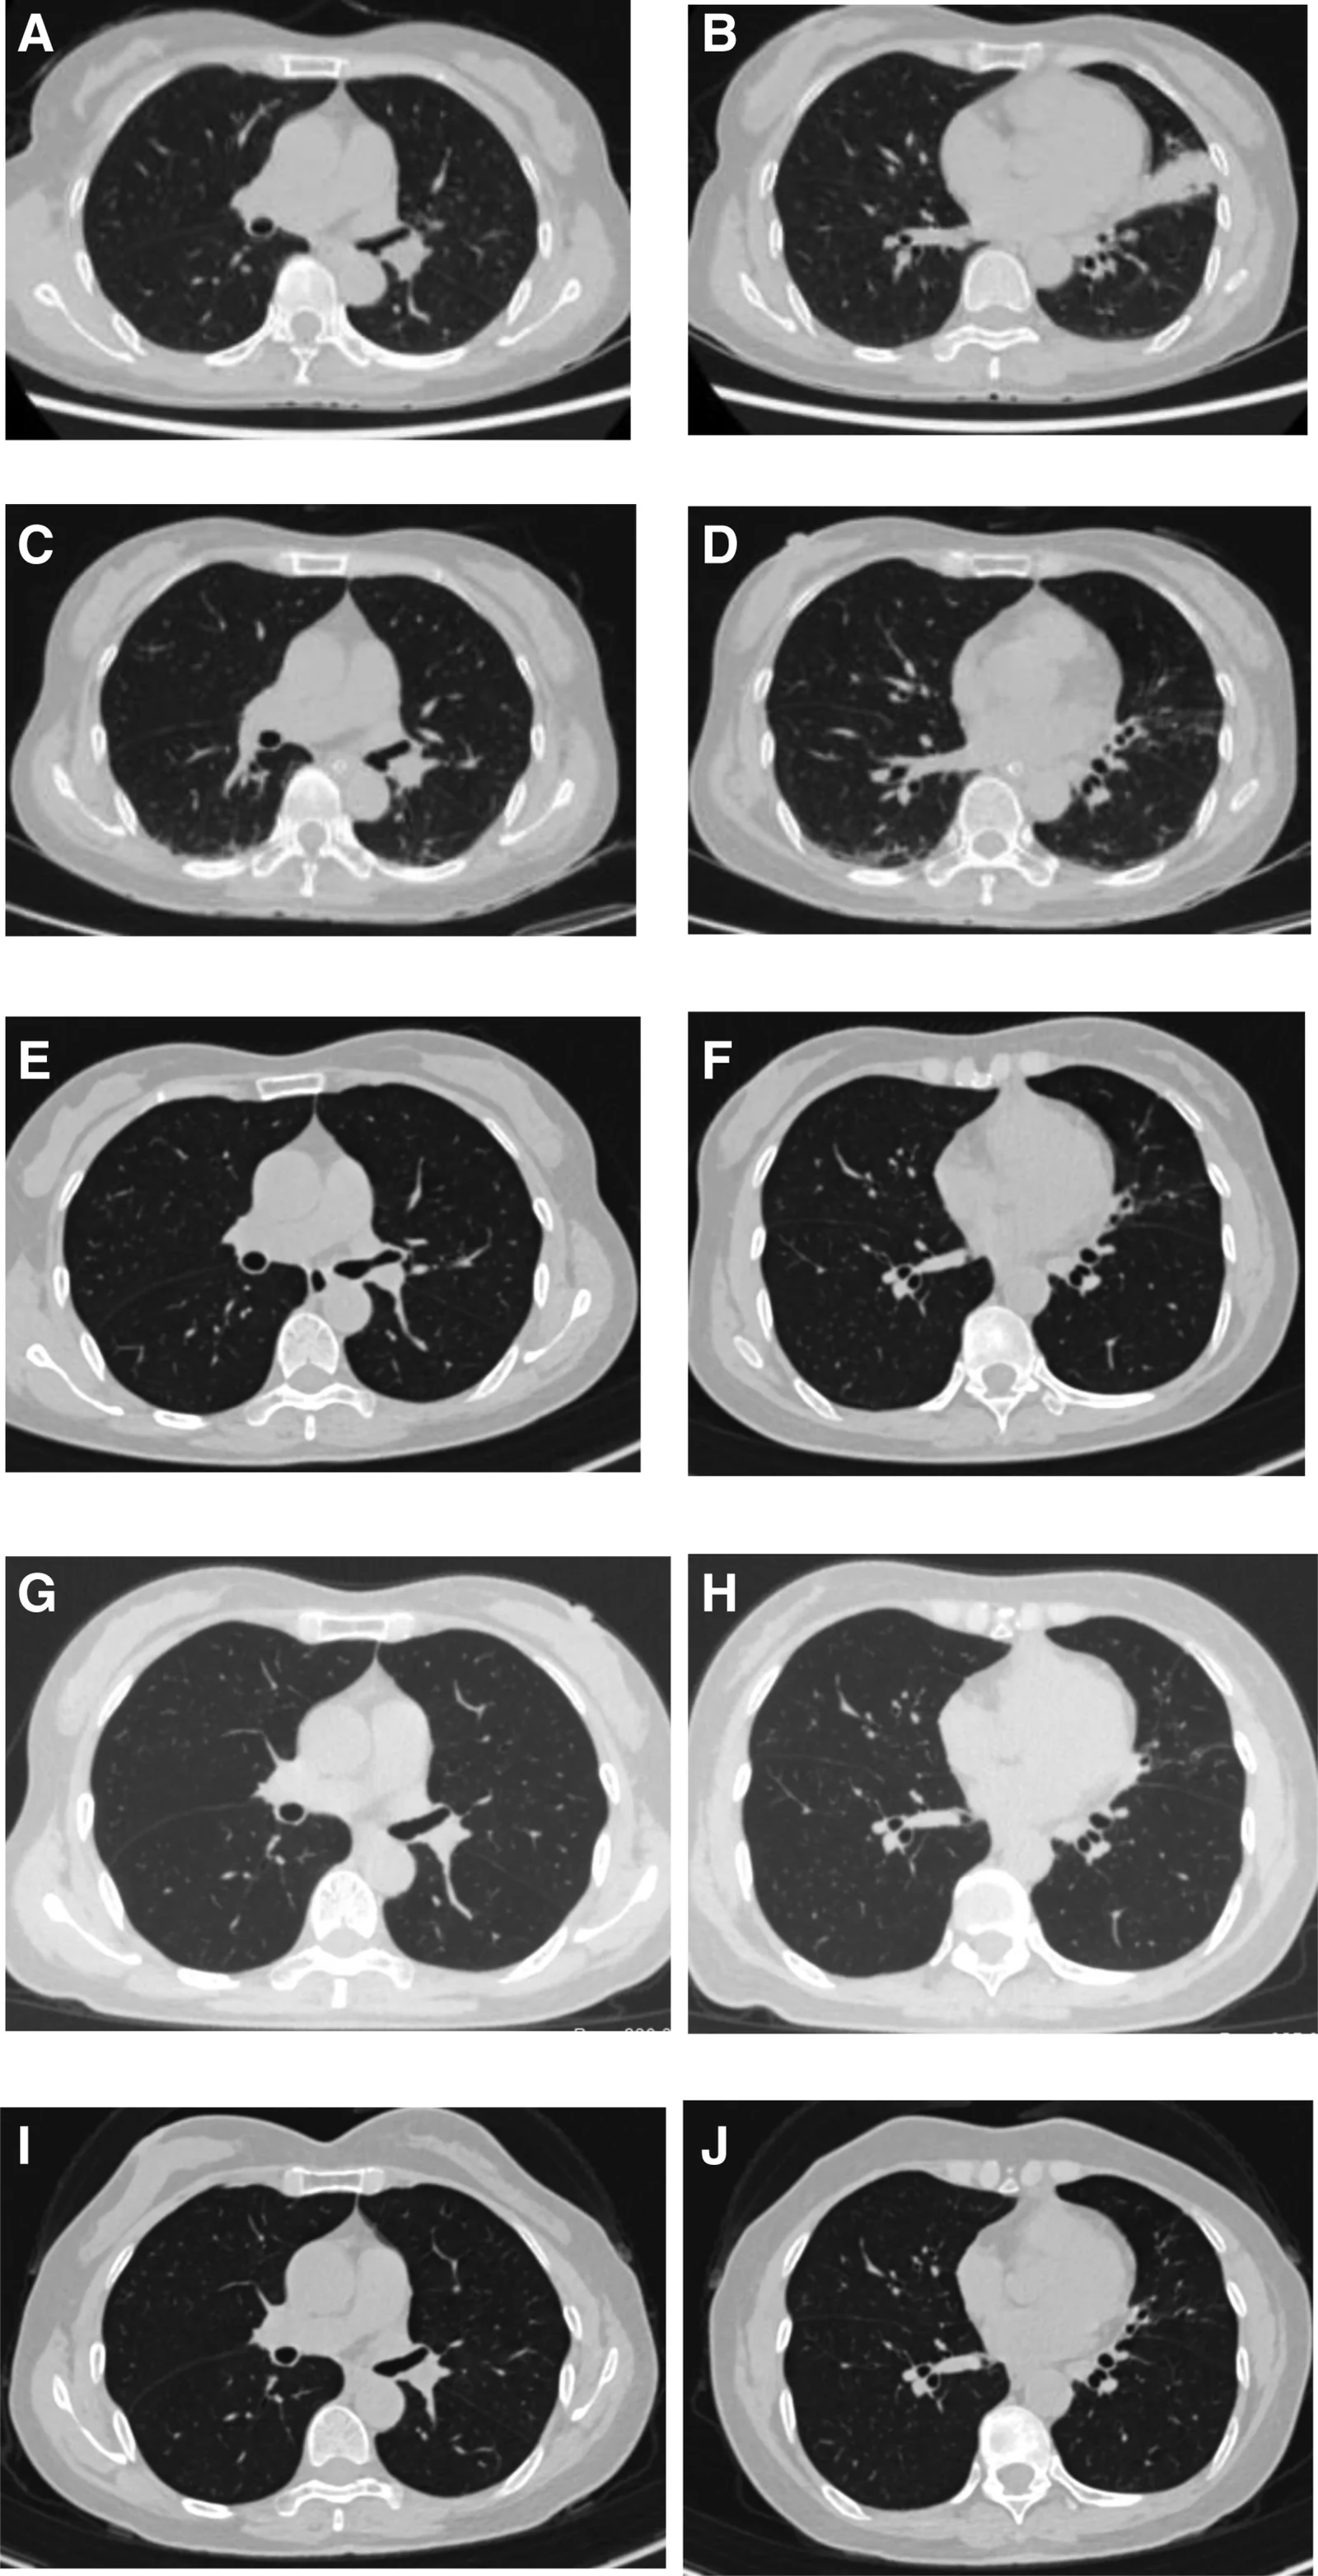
(A) and (B) The Chest CT scans at 2-week treatment. (C) and (D) The Chest CT scans at 1-month post-discharge. (E–H) and (I–J) Chest CT scans at 8, 18, and 36 months after discharge.
